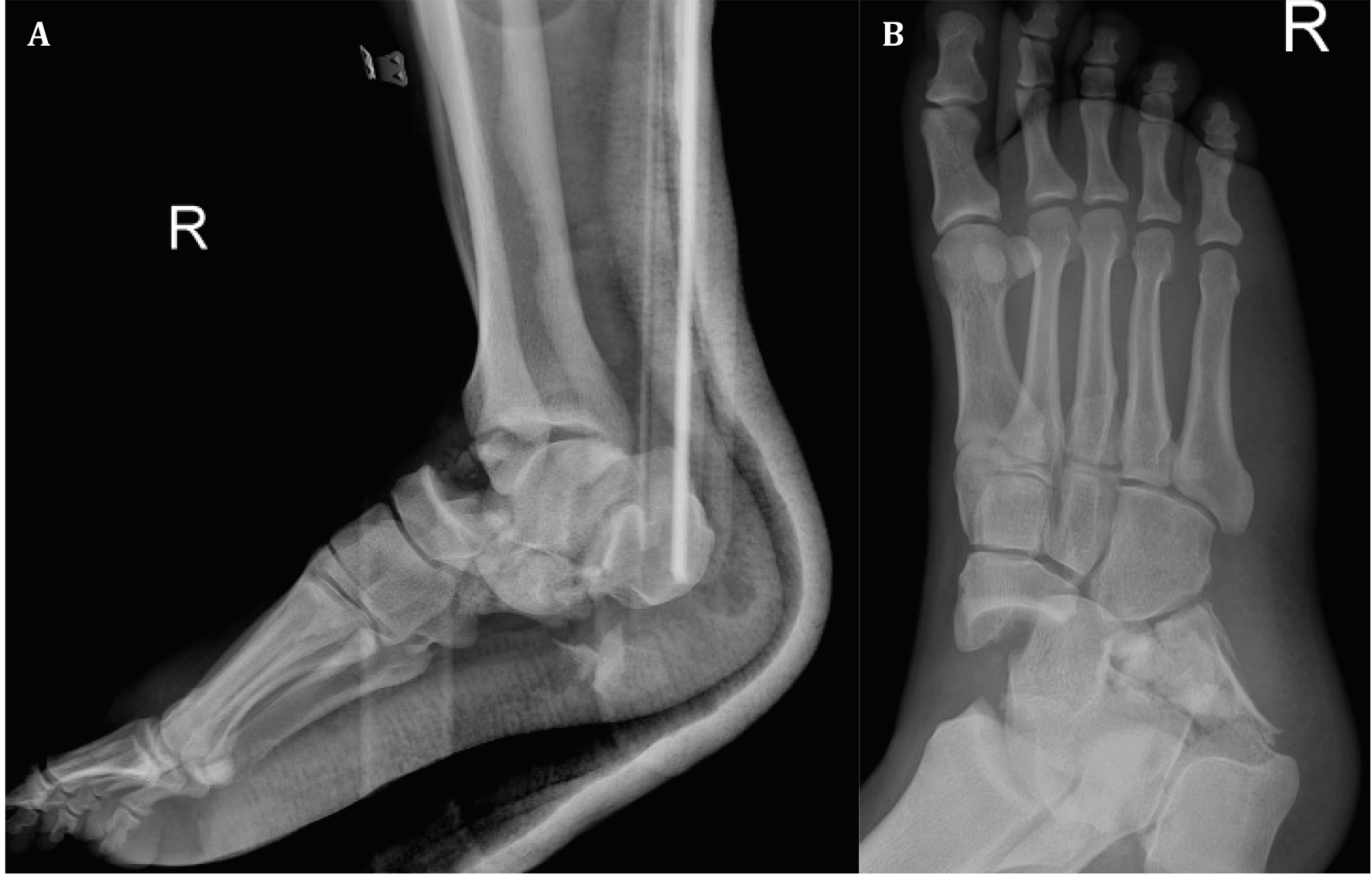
(A) Lateral of right ankle and (B) oblique of right foot radiographs revealing dislocated talonavicular joint with displaced calcaneal fracture and multiple fragments in the plantar area
